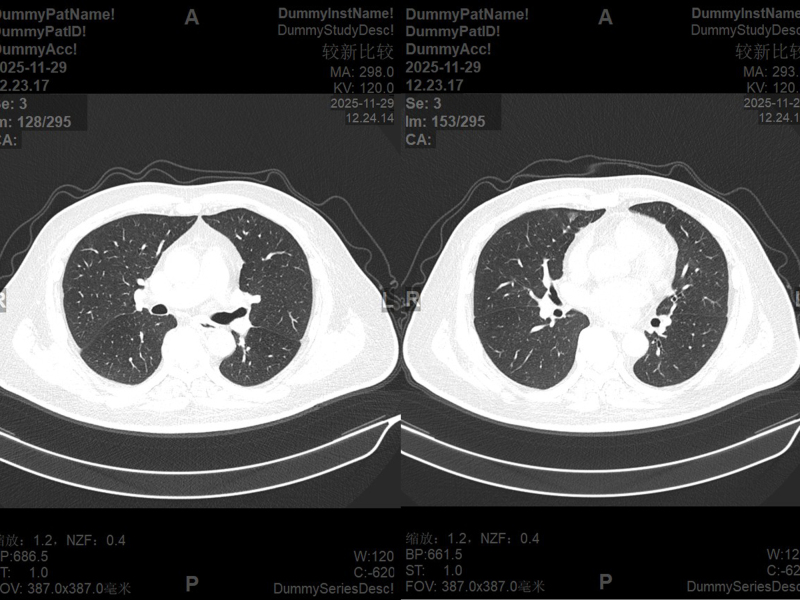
The chest CT on the 15th day after discharge showed that the inflammatory lesions had been completely absorbed. CT = computed tomography.
